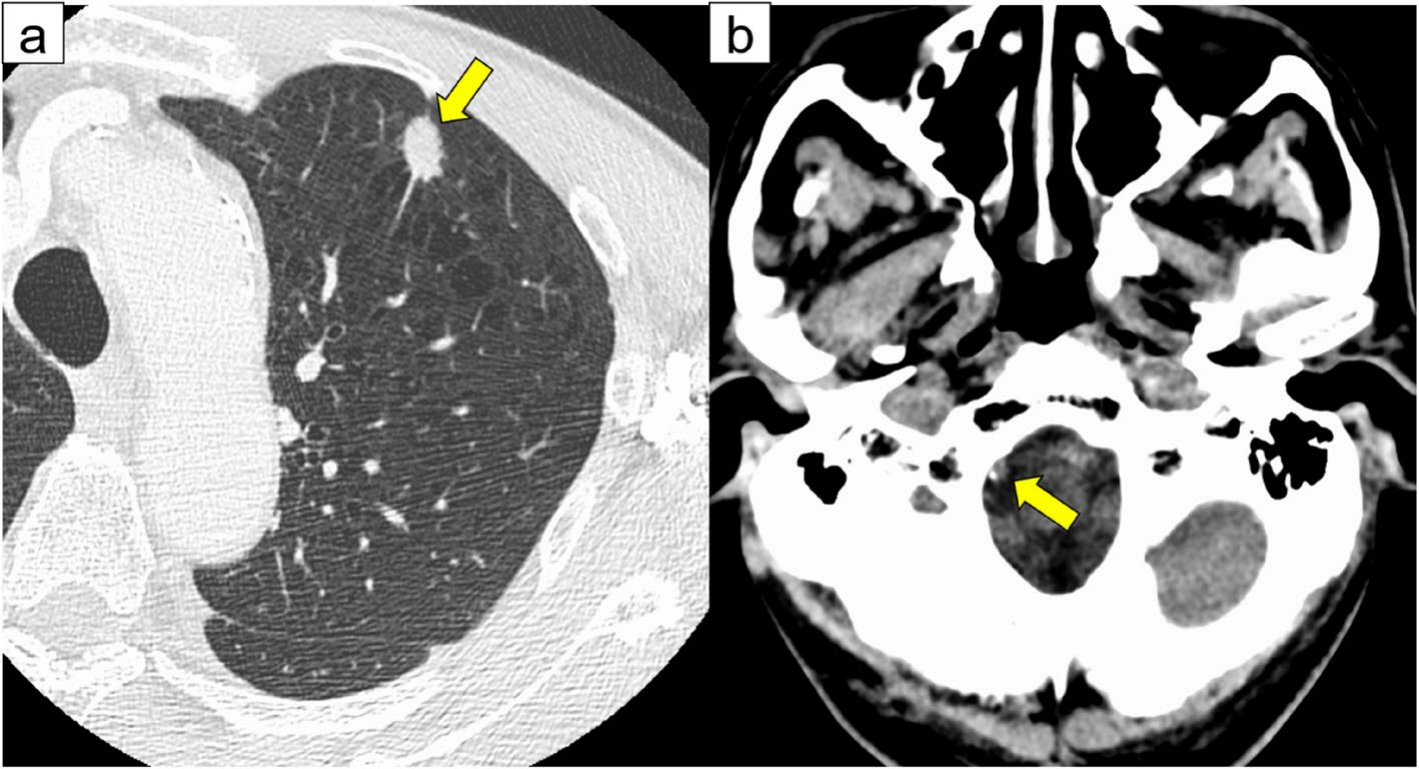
a Chest computed tomography scan showing a tumor in the left upper lobe of the lung (yellow arrow). b Head computed tomography scan showing slight calcification in the right vertebral artery (yellow arrow)
